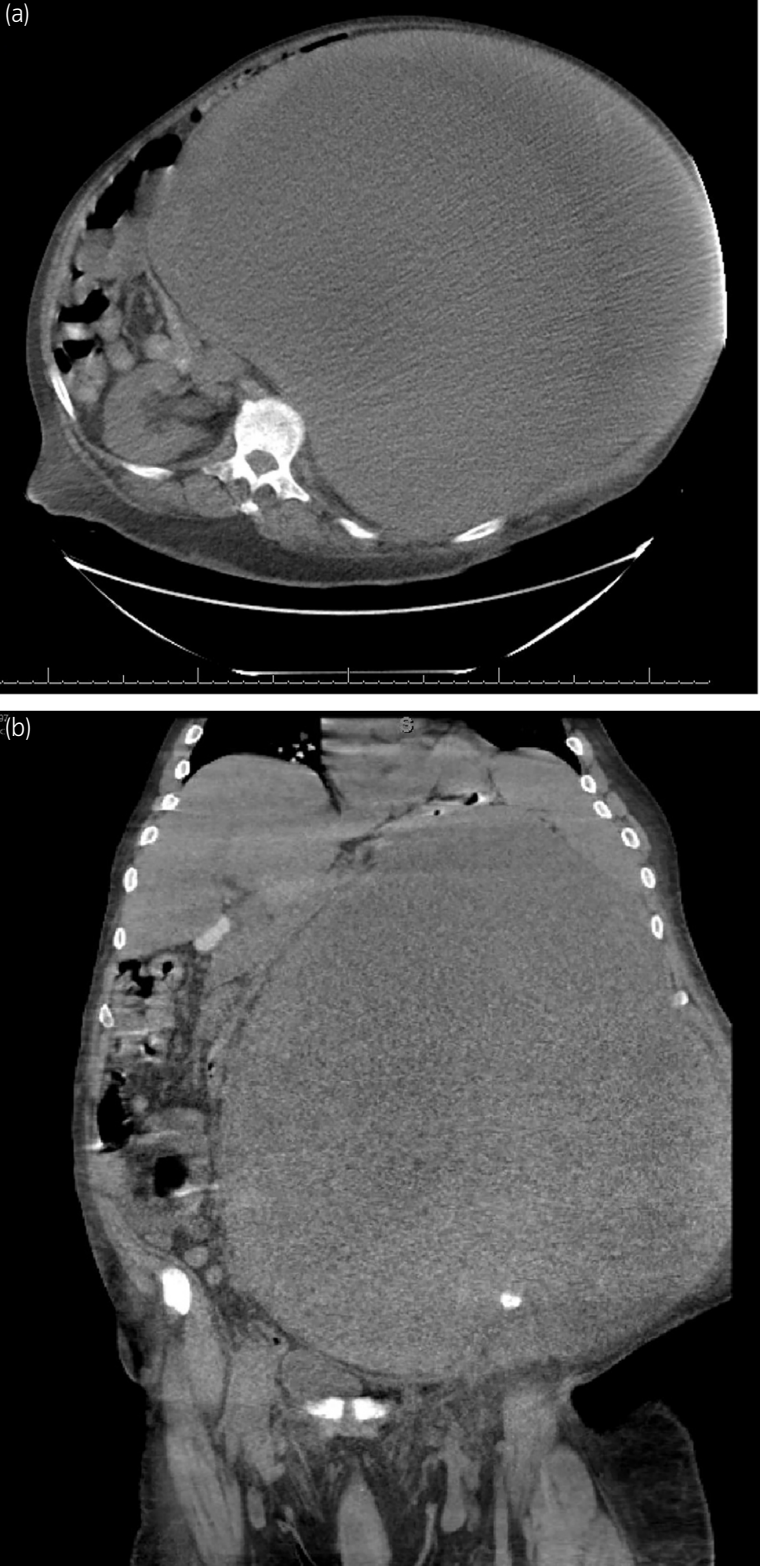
Abdominal CT images (a: axial view, b: coronal view) revealed a huge mass occupying most of the abdomen and pelvis.
